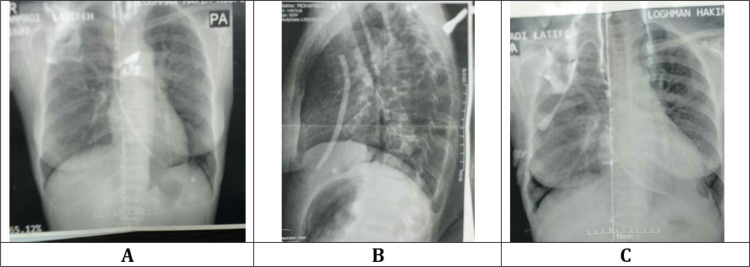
Posterior-anterior (A), Lateral (B), and anterior-posterior (C) views of patient's chest x-ray
